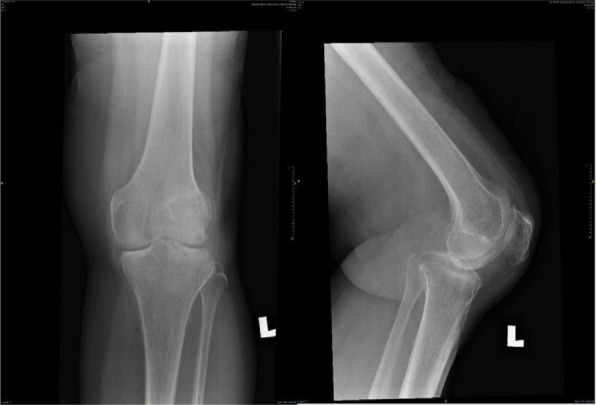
Radiographs AP and lateral views of the left knee, 7 months post-arthrotomy and antibiotic therapy, shows some reformation of the medial femoral and tibial condyles.
